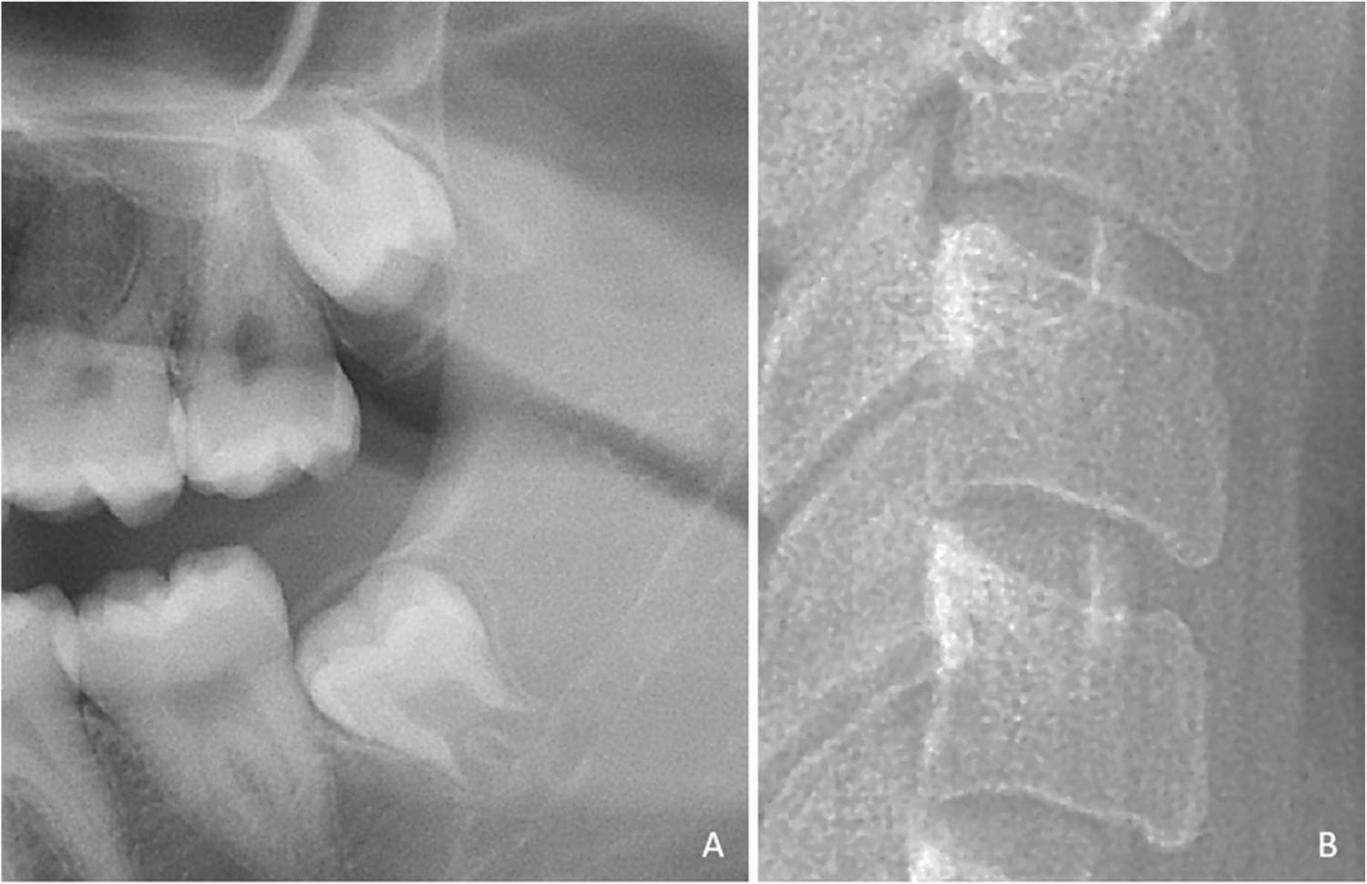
Example of a female individual with chronological age 17.76 years. The cropped panoramic radiograph (A) shows the left third molars and the cropped cephalometric radiograph (B) shows the inferior surfaces of cervical vertebrae C2, C3, and C4. The maxillary third molar was classified as stage E and the mandibular third molar was classified as stage F. The anterior inferior apophysis ossification stages of cervical vertebrae C2, C3, and C4 were all classified as stage 4
